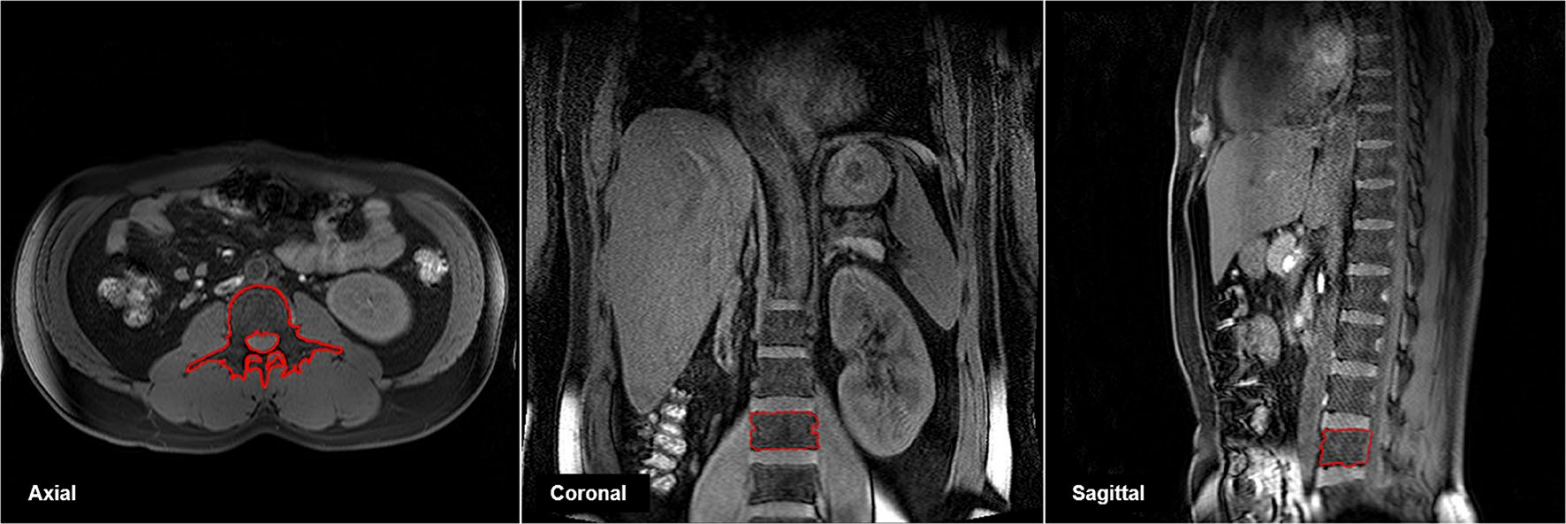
Abdominal MR images at third lumbar spine (L3, inside red-line) level.
